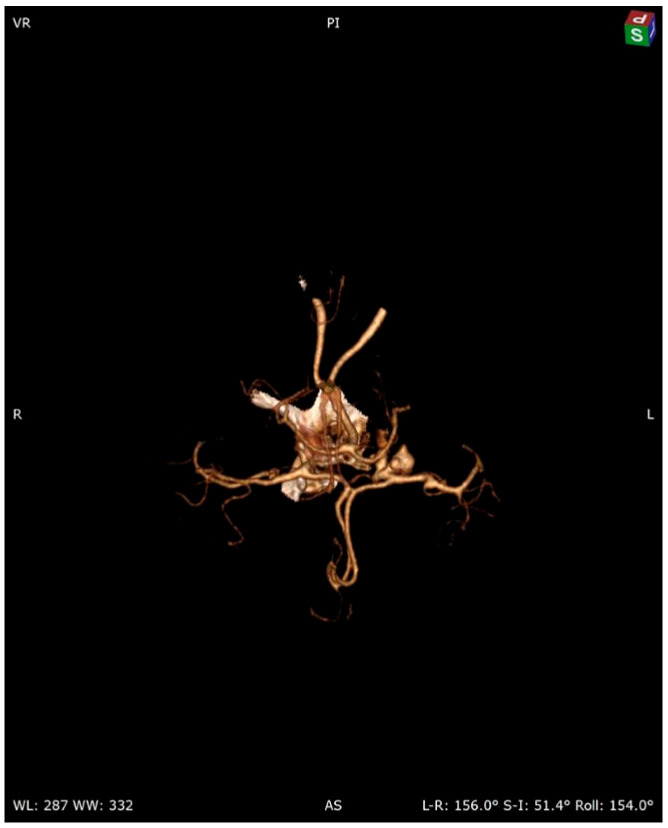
Cerebral 3D CT angiography.
